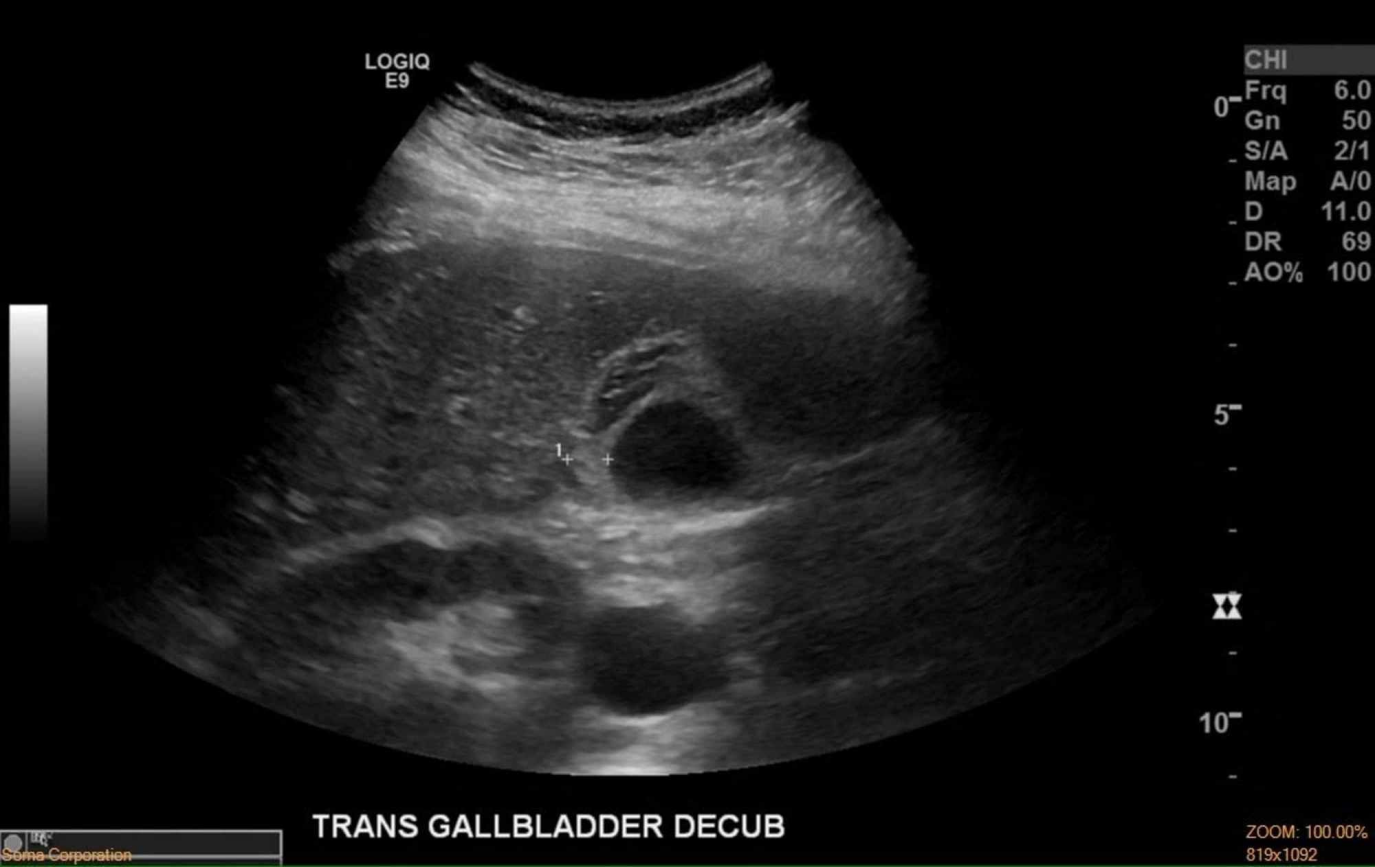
Gallbladder US showing 1.0 cm (10 mm) gallbladder wall thickening without the presence of gallstones or sludge cm = centimeters; US = ultrasound
